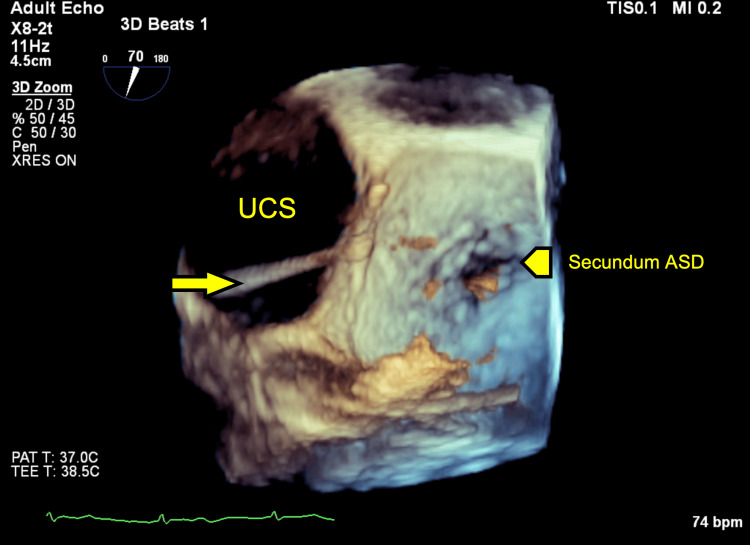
Intraprocedural 3D TEE, LA aspect view, diastolic phase, showing the IAS with ASD secundum (arrowhead) and demonstrating a multipurpose catheter (arrow) passing through the UCS. TEE: Transesophageal echocardiography; LA: Left atrium; IAS: Interatrial septum; ASD: Atrial septal defect
